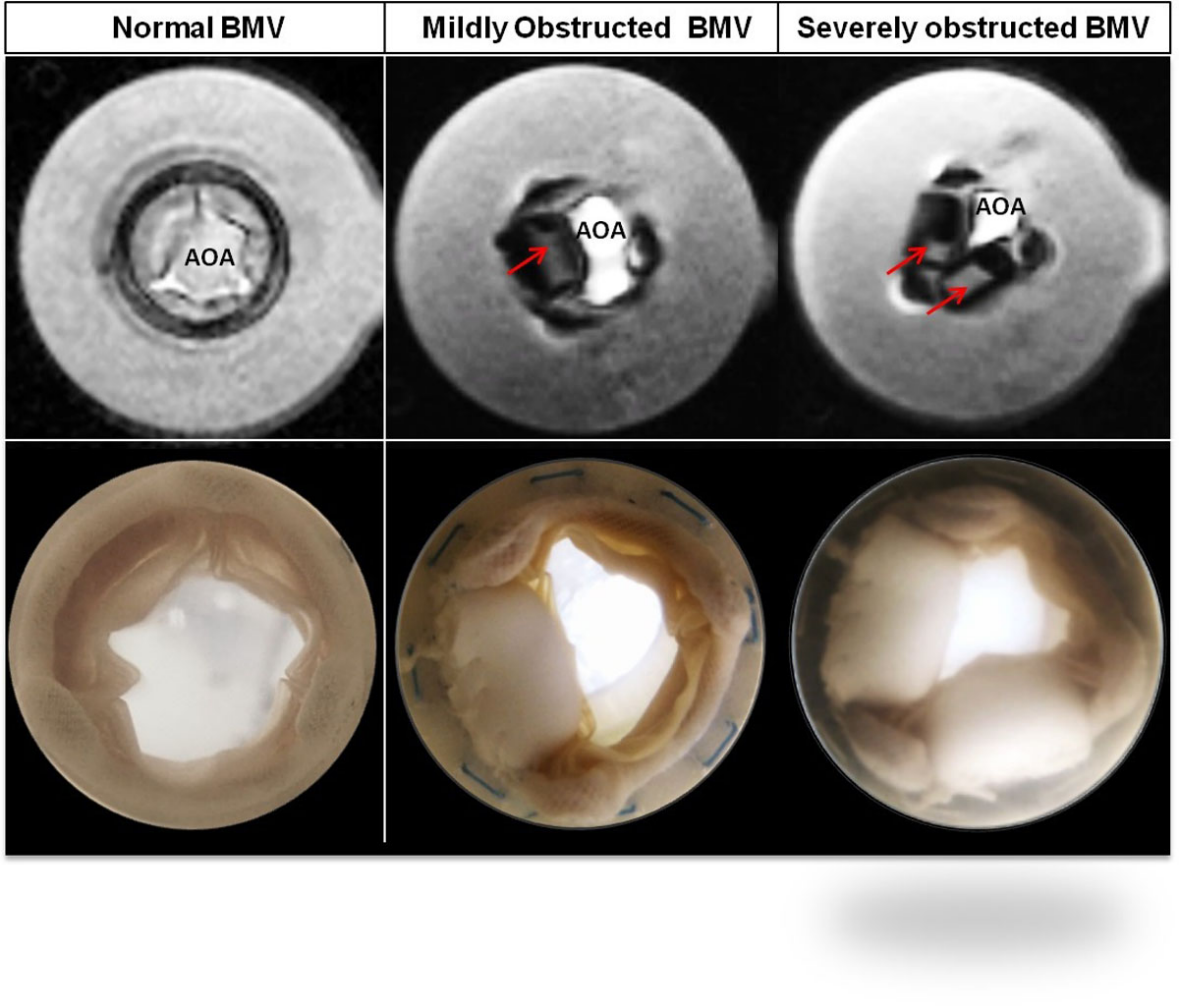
# Cardiac MRI functional assessment of bioprosthetic mitral valves: an in vitro comparison against doppler echocardiography
